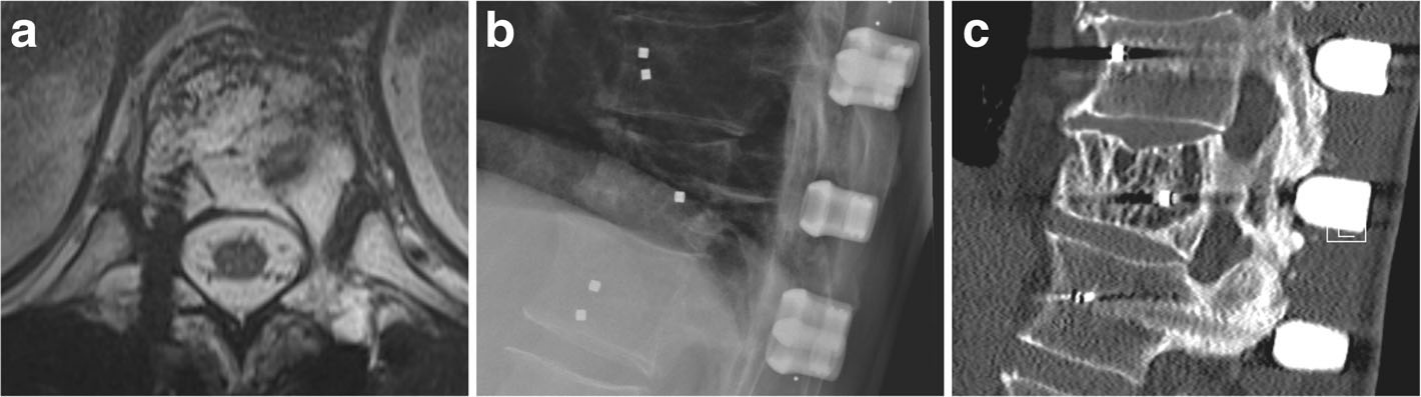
The postoperative imaging provides excellent assessability of the bony anatomy despite proximity of the implants. The implants are best depicted in the MRI with artefact reduction protocol (a, b). The last follow-up CT scan shows the accomplished posterolateral fusion (c)
